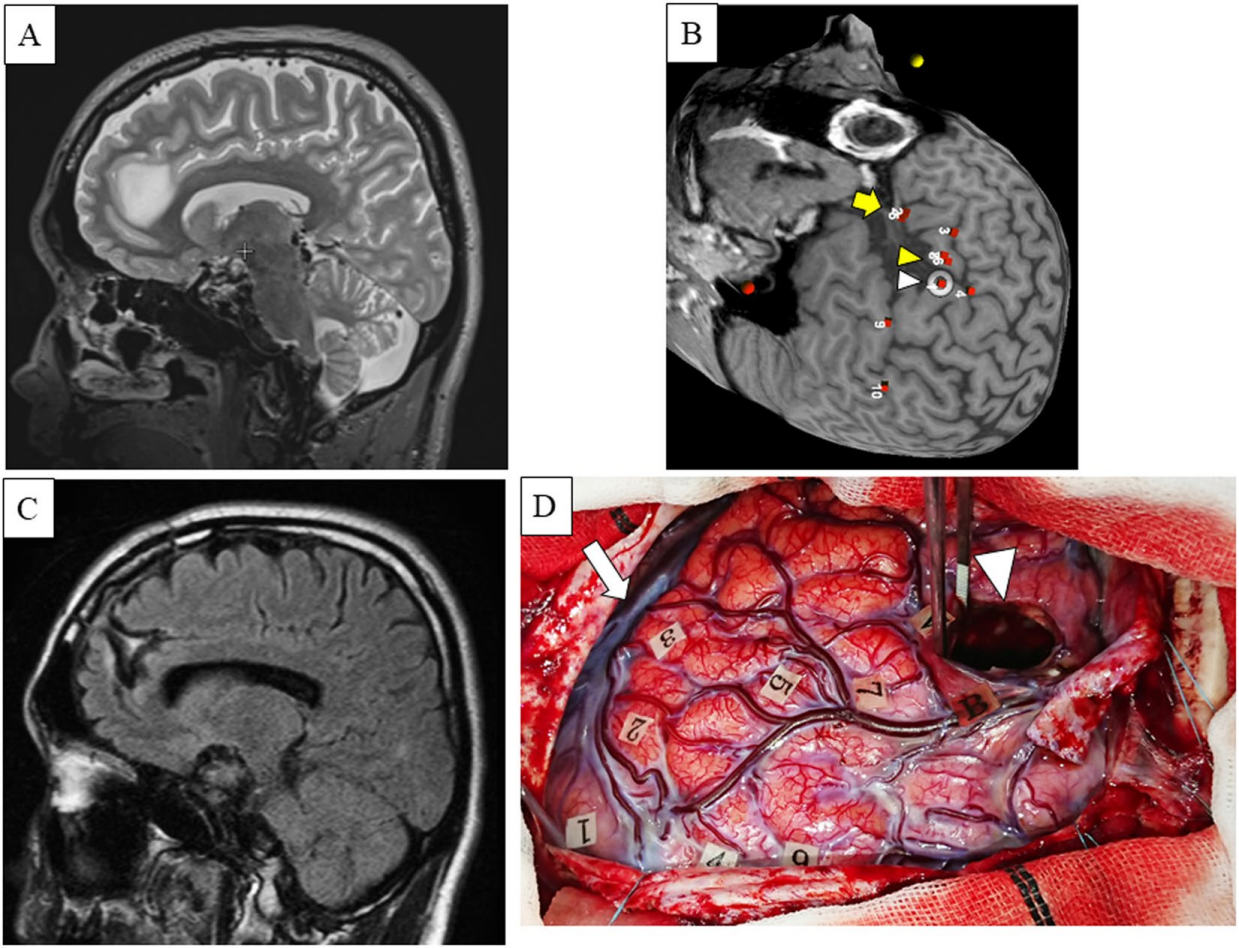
(A) Representative image of patient 44. Preoperative axial fluid-attenuated inversion recovery-weighted magnetic resonance images showing a left frontal low-grade glioma (left cingulate gyrus) in a 31-year-old right-handed man with no relevant medical history who presented with episodic headache. (B) Preoperative three-dimensional images obtained with navigated repetitive transcranial magnetic stimulation (nrTMS) language mapping using the picture-naming task. Anomia was induced in the precentral gyrus (white arrowhead) and in the opercular part of the inferior frontal gyrus (yellow arrowhead). Phonemic paraphasia was induced in the triangular part of the inferior frontal gyrus (yellow arrow). These responses correspond to the intraoperative image (Fig. 4D) showing cortical mapping with direct cortical stimulation (DCS). (C) Postoperative axial fluid-attenuated inversion recovery-weighted magnetic resonance imaging showing gross-total resection of the mass using awake brain mapping. (D) Intraoperative image obtained after resection showing letter tags that indicate tumor boundaries (A–B). Stimulation over the precentral gyrus induced speech arrest (number tag: 1); stimulation over the opercular part of the inferior frontal gyrus induced speech arrest (number tag: 2); stimulation over the triangular part of the inferior frontal gyrus induced semantic paraphasia (number tag: 3). The dorsolateral prefrontal cortex (DLPFC) was mapped on the lateral tumor side (number tags: 5, 7), using a 5-digit backward digit span task to confirm verbal working memory. Furthermore, positive responses were detected using a 4-digit backward digit span task (number tags: 4, 9); arrowhead, corridor; arrow, sylvian fissure.
